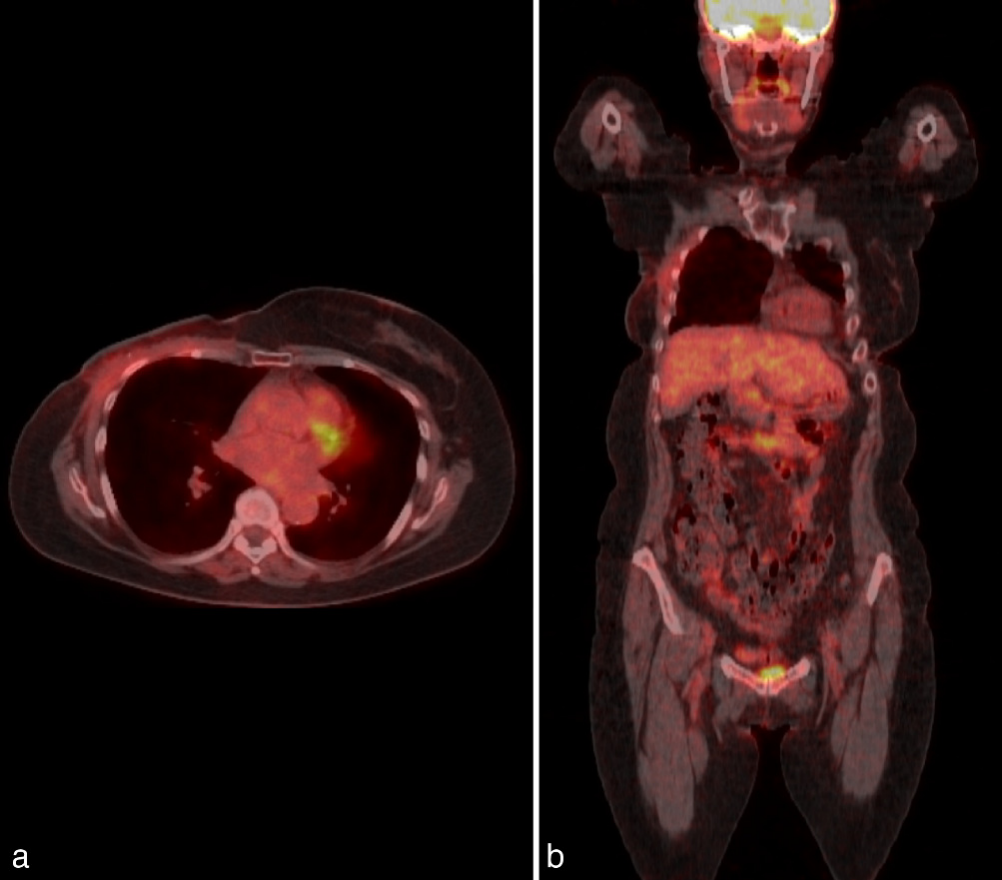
(a) Axial and (b) coronal views of ^18^F-fludeoxyglucose positron emission tomography-CT performed 3 months postoperatively demonstrating reduction of previous 18F-fludeoxyglucose uptake.
